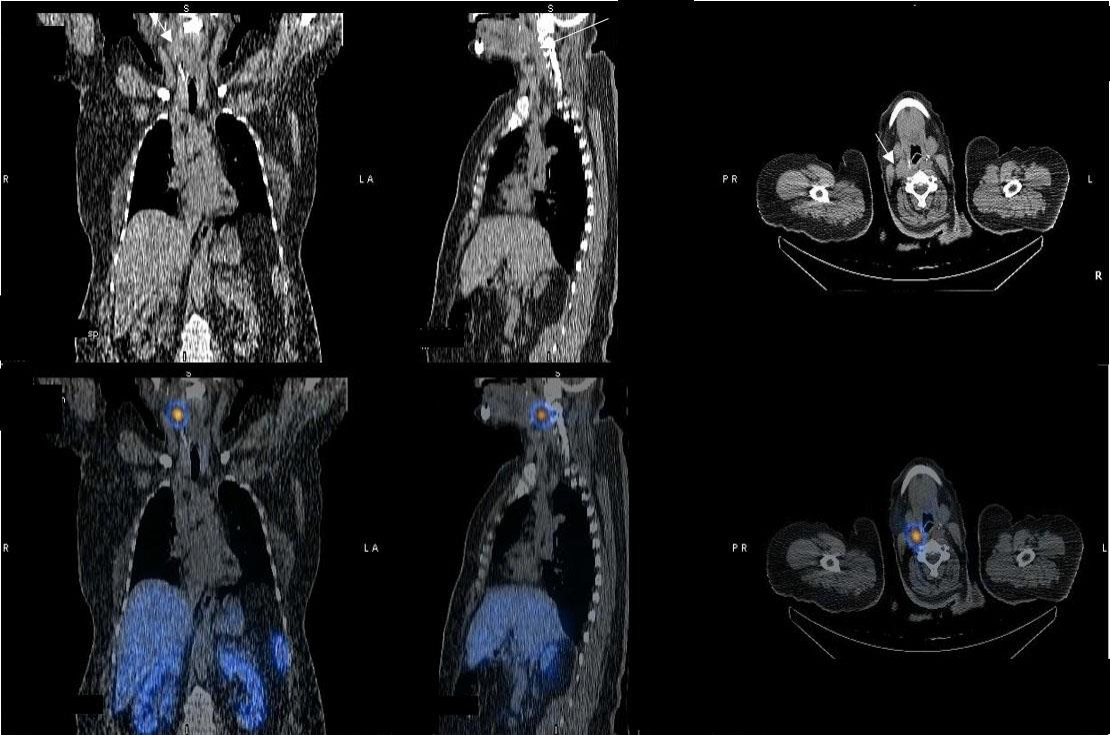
SPECT/CT images localized the Octero avid lesion to a mass in the right cervical level II
